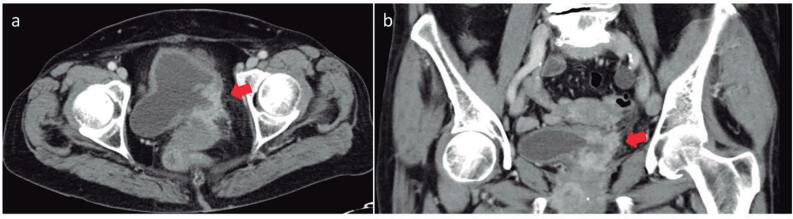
Morphological characteristic of locally advanced tumor stage in CT scan Representative pelvic CT scan showing locally advanced tumor stage in transversal plane (A) and coronal plane (B).
